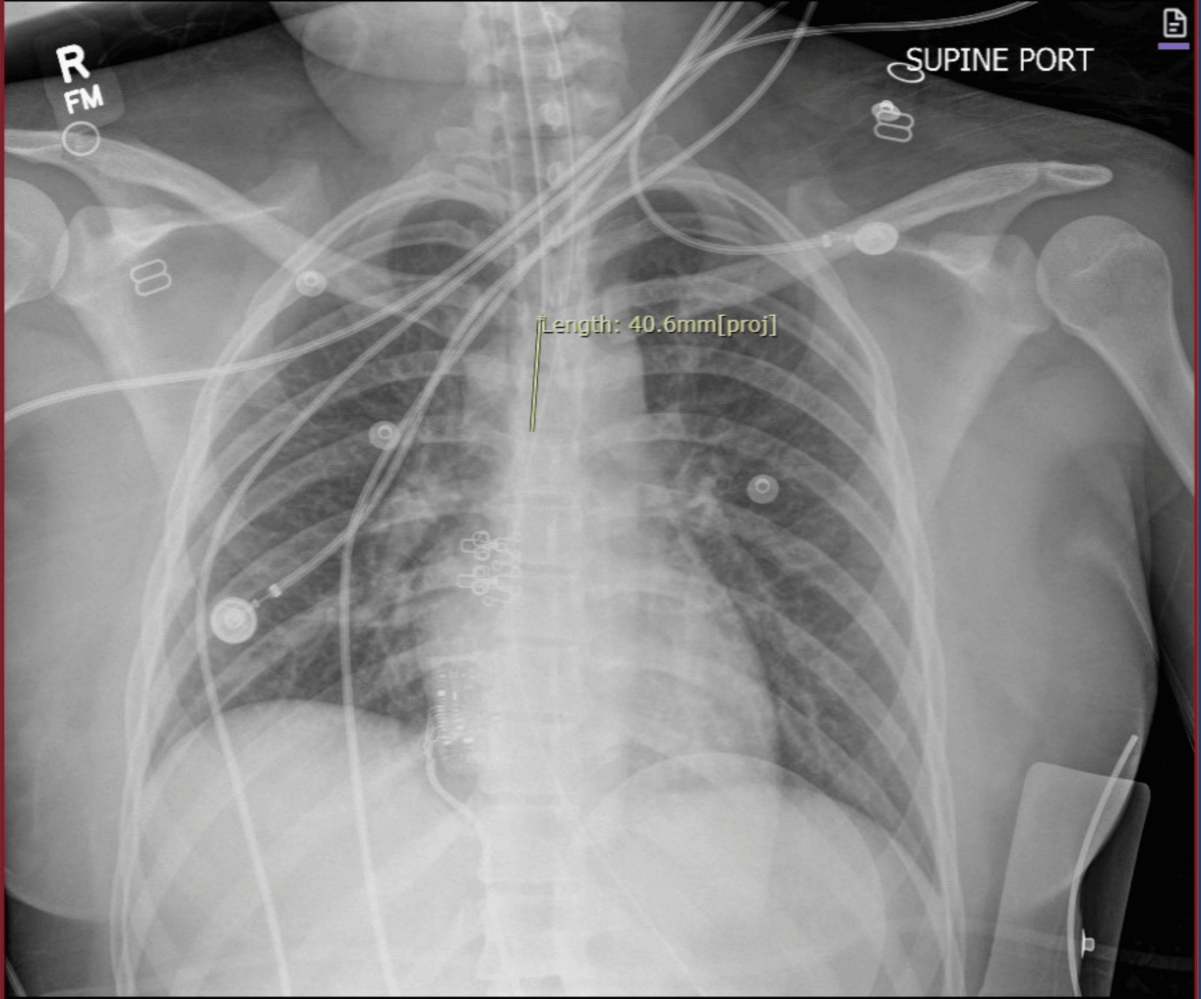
Chest X-ray of a patient with poppers-induced methemoglobinemia after intubation showed no acute abnormality
